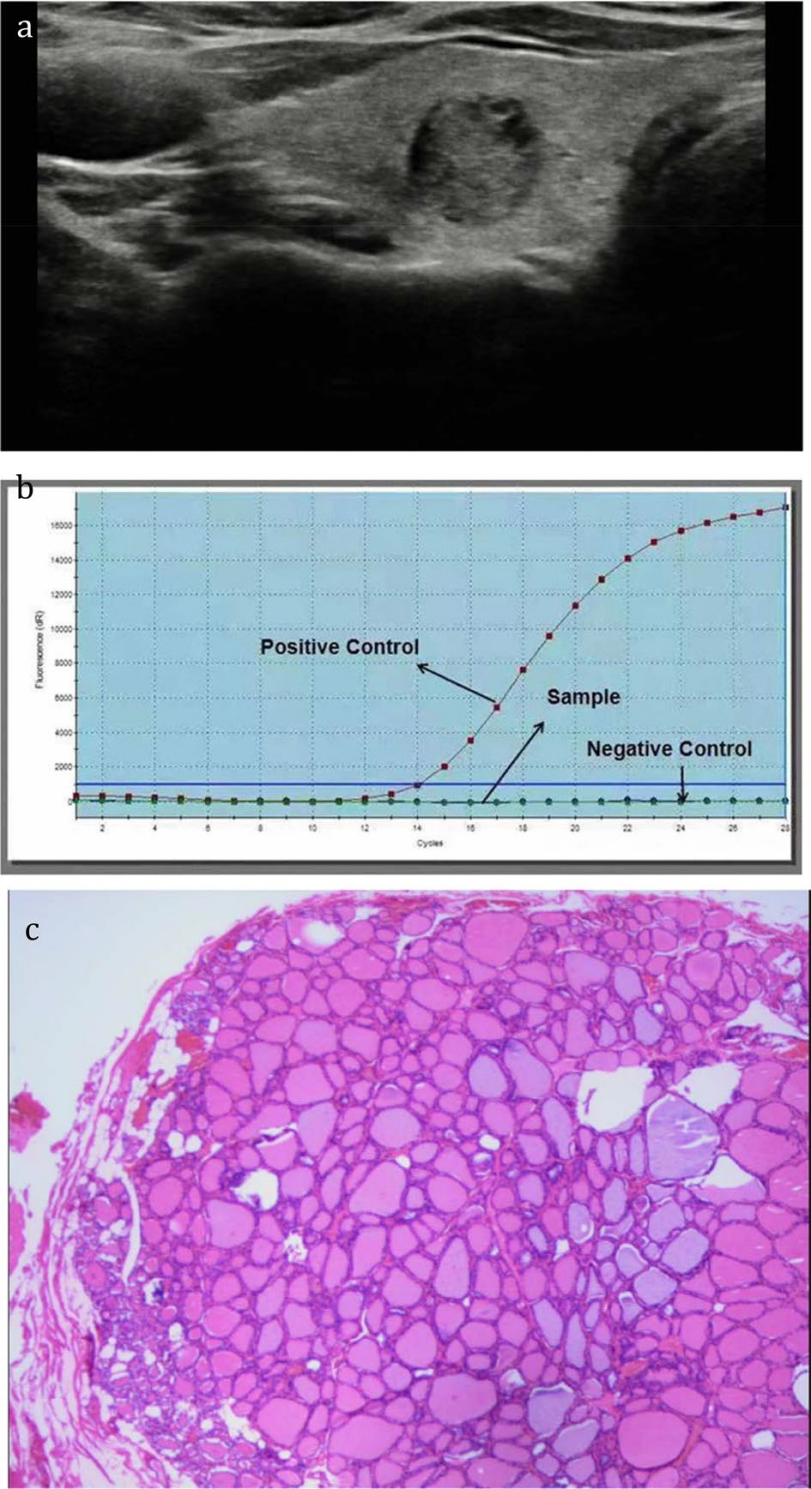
A thyroid nodule, assigned to Bethesda category III, was considered benign diagnosed by the combination of TIRADS and BRAF^V600E^ analysis. The nodule proved to be a benign one confirmed by postoperative pathology. (a), the 2D sonogram classified as TIRADS 4a, (b), wild-type BRAF^V600E^ gene, (c), nodular goiter confirmed by postoperative pathology.
